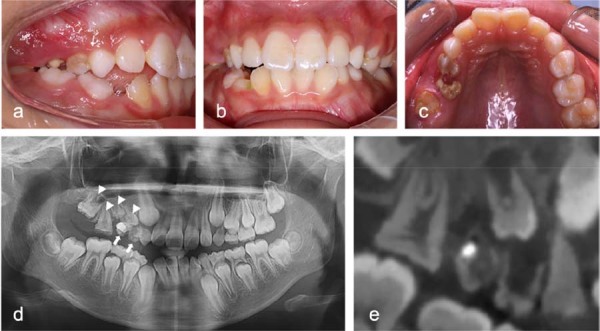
Radiographic and clinical presentation of a 9-year-old girl with ROD in the maxillary right quadrant. (a-c) Intraoral findings: (a) Buccal view showing severe caries and gingival inflammation associated with teeth 54 and 55. (b) Frontal occlusal view showing the overall dental status. (c) Palatal view highlighting the hypoplastic and yellowish discoloration of tooth 16, a characteristic ROD feature. (d, e) Radiographic evaluation: (d) Panoramic radiograph and (e) CBCT scan revealing root resorption and periapical radiolucency in primary teeth 54 and 55 (indicated by arrows). Permanent teeth 14 to 17 exhibit reduced crown size, thinner enamel and dentin layers, lower mineral density, and delayed developmental stages compared with their contralateral counterparts.
